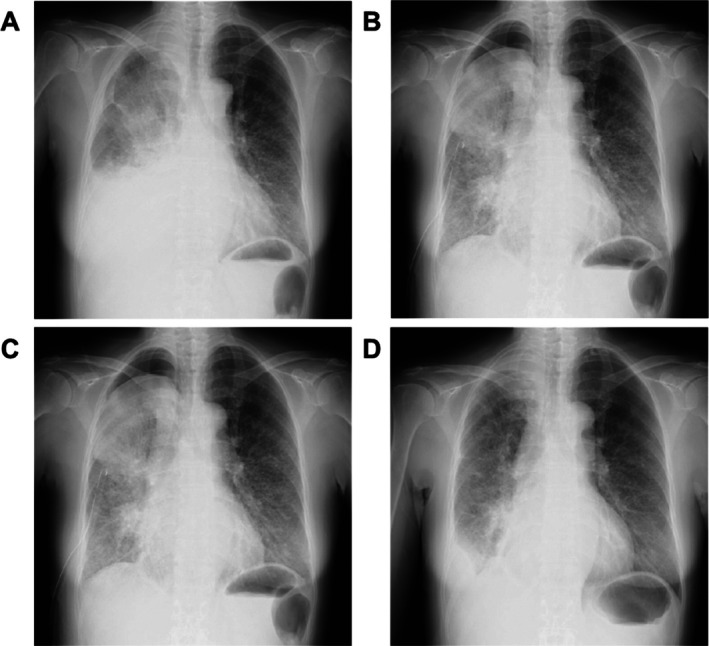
A representative case of serial frontal plane chest radiographs. This series depicts the lungs of a 50‐year‐old woman with lung adenocarcinoma. (A) Before tube thoracostomy; (B) immediately before intrapleural hypotonic cisplatin administration; (C) at 4 weeks; (D) at 8 weeks post‐administration. The LES score was two points pre‐thoracostomy, subsequently decreased to 1.5 just before the intrapleural administration of hypotonic cisplatin, and further decreased to 1 at 4 weeks post‐administration. LES, lung expansion scale.
